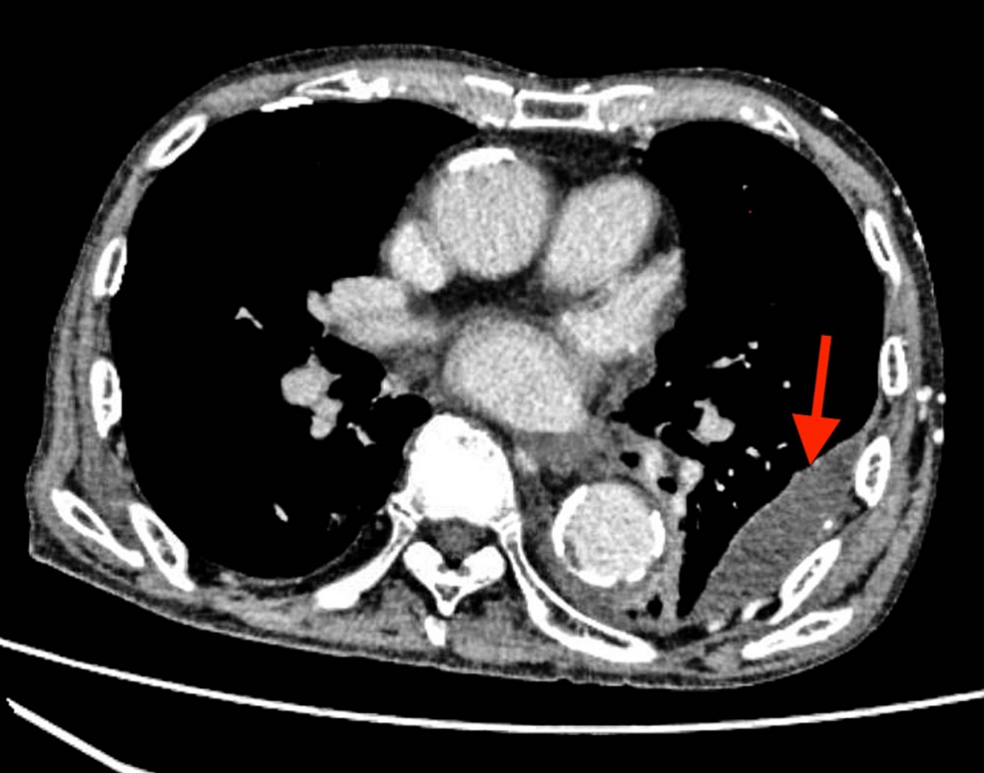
CT findings showing increased pleural effusion and pleurisy (red arrow)
